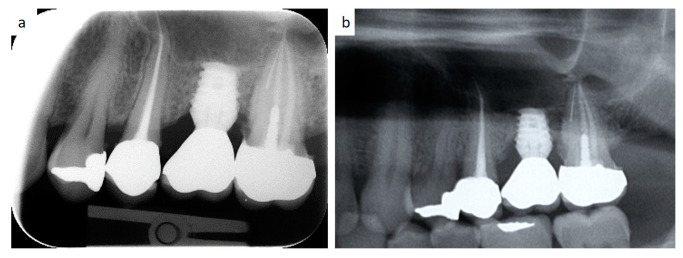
Periapical radiograms showing the bone loss at a 2-year (a) and a 10-year follow-up (b) around a short spiral implant (6.25 × 4.2 mm in diameter) supporting a single metal–ceramic crown.
